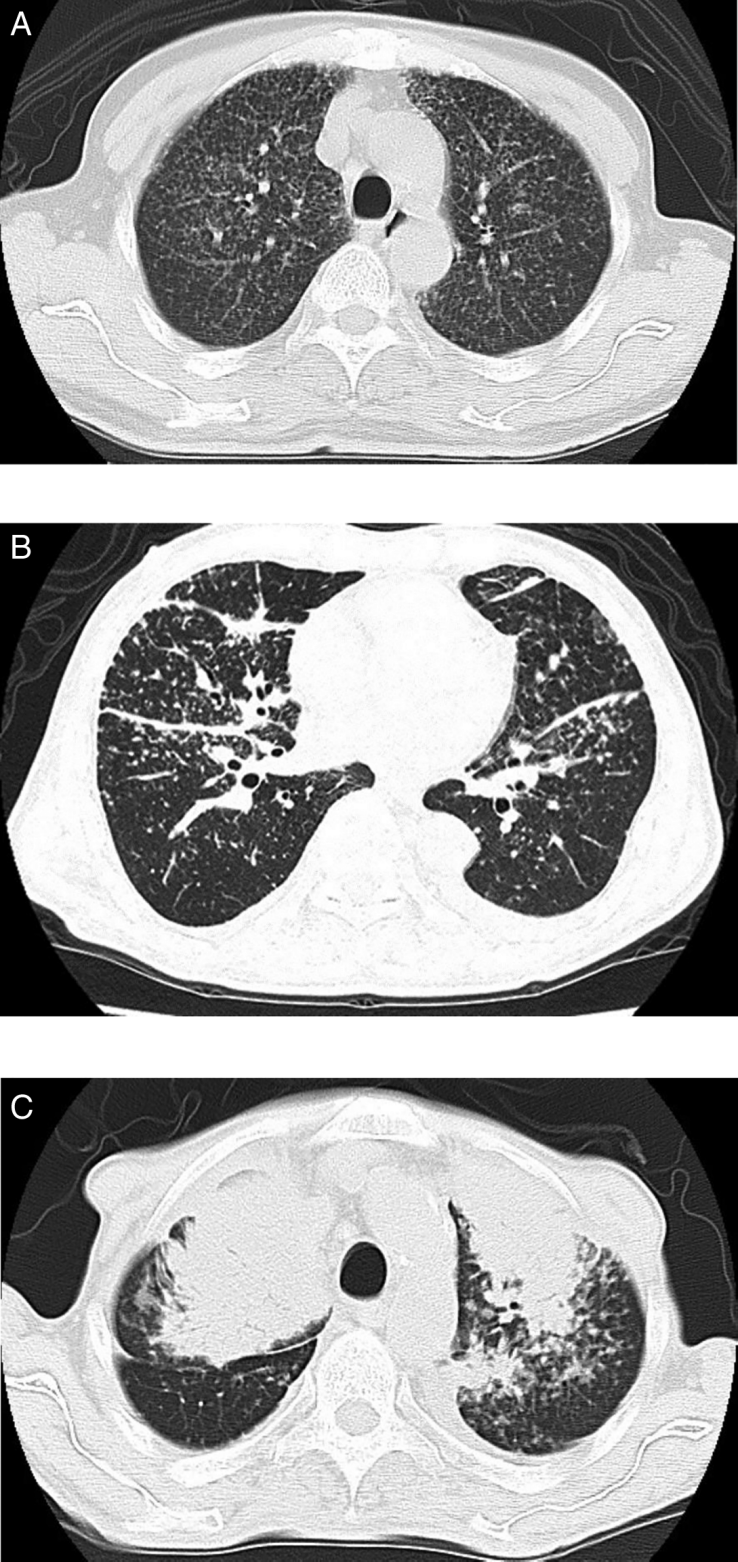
(A) High‐resolution computed tomography of the lung showed small diffuse nodules, randomly distributed throughout lung fields. (B) Chest computed tomography (CT) taken a few months after the initial CT showed thickening of interlobular septa and interlobar fissures, as well as small nodules. A small amount of bilateral pleural effusion was also observed. (C) Chest CT showed several large tumours in the bilateral lung region, in addition to small nodules. Pleural effusion is observed in the left thoracic cavity.
